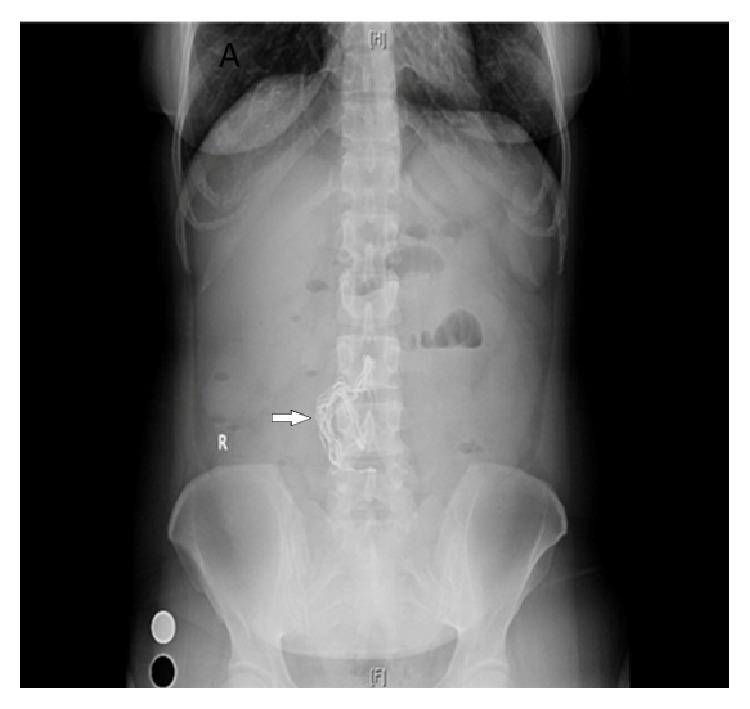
A direct abdominal radiography showed multiple opaque substances accumulated as strips in the mid-line.
